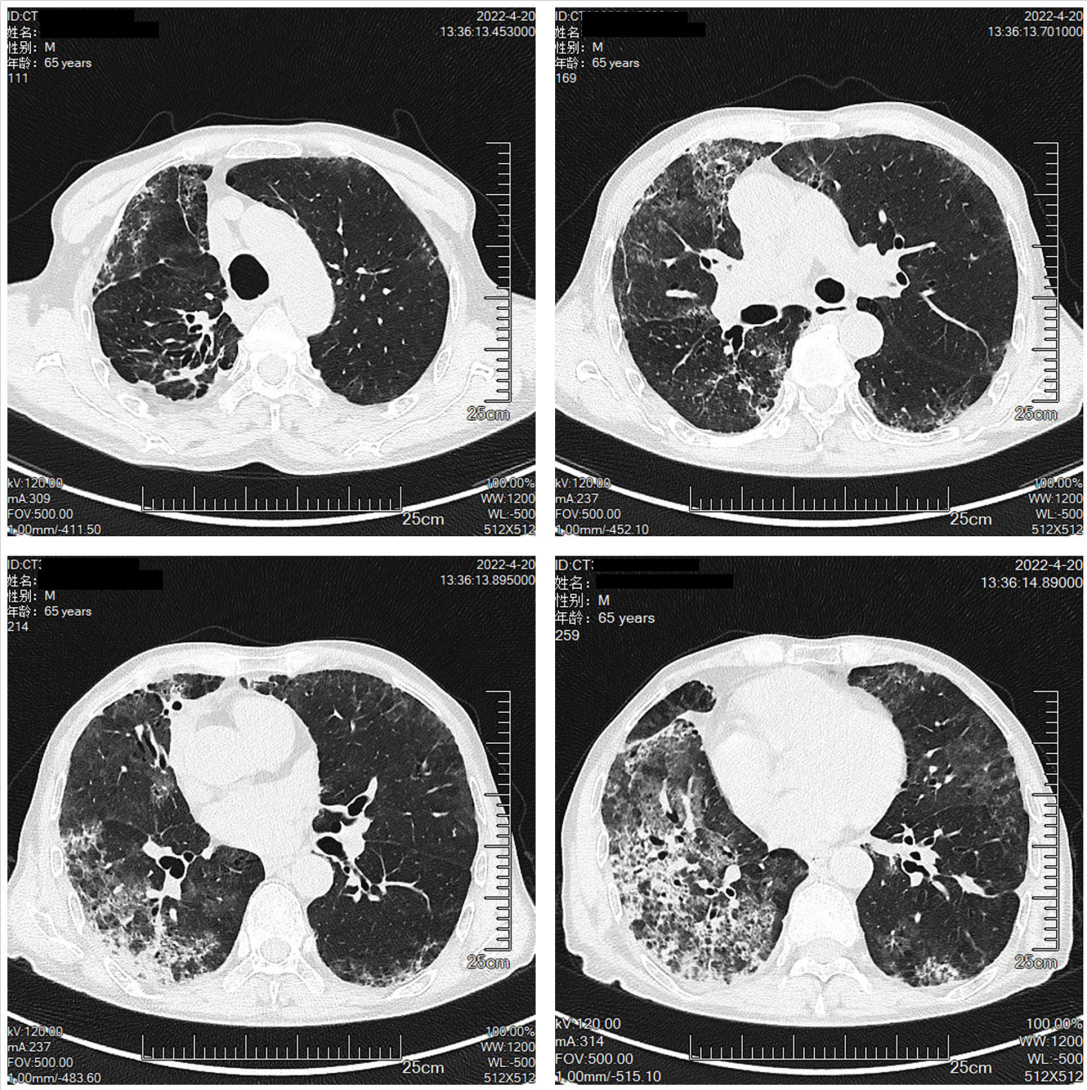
The Chest CT on April 20th.
